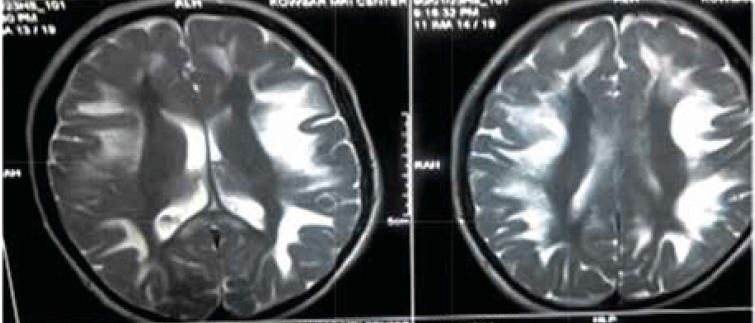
T2-weighted views of brain MRI shows high-intencity signals in periventricular white matter and midbrain
